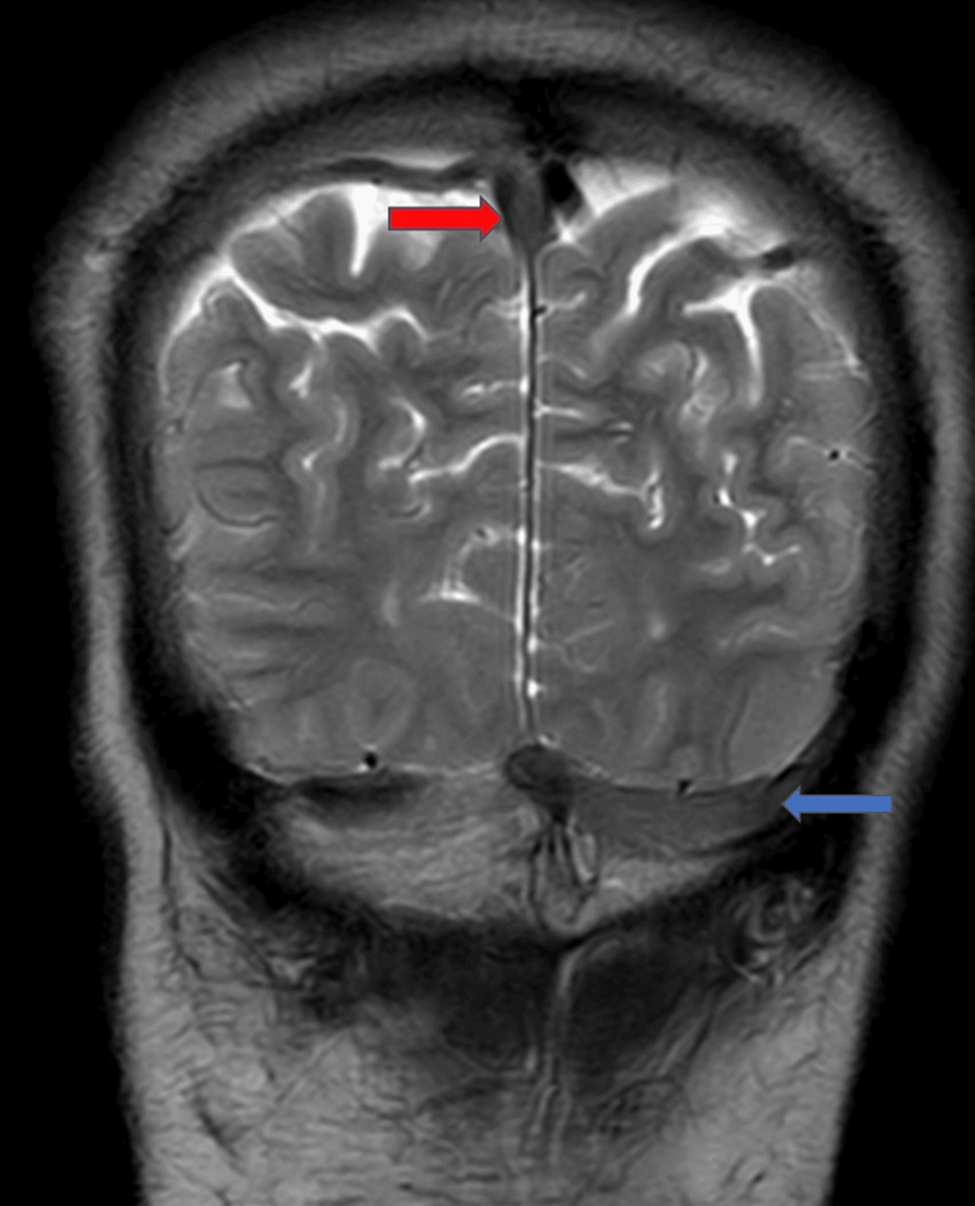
MRI of the brain showing loss of normal signal intensity in the superior sagittal sinus (red arrow) and transverse sinus (blue arrow)
